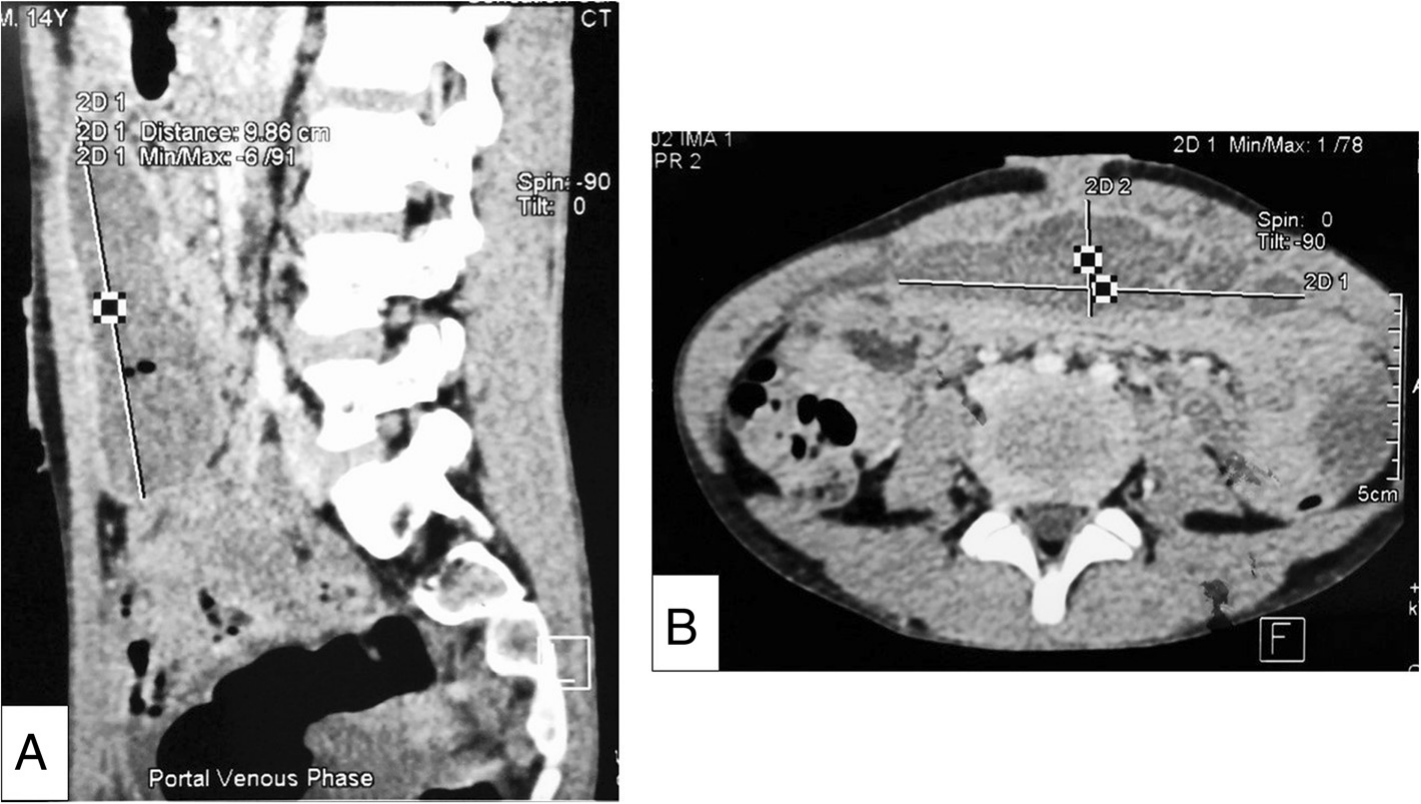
Computed tomography scan of abdomen showing the urachal abscess. a Sagittal view b Transverse view
